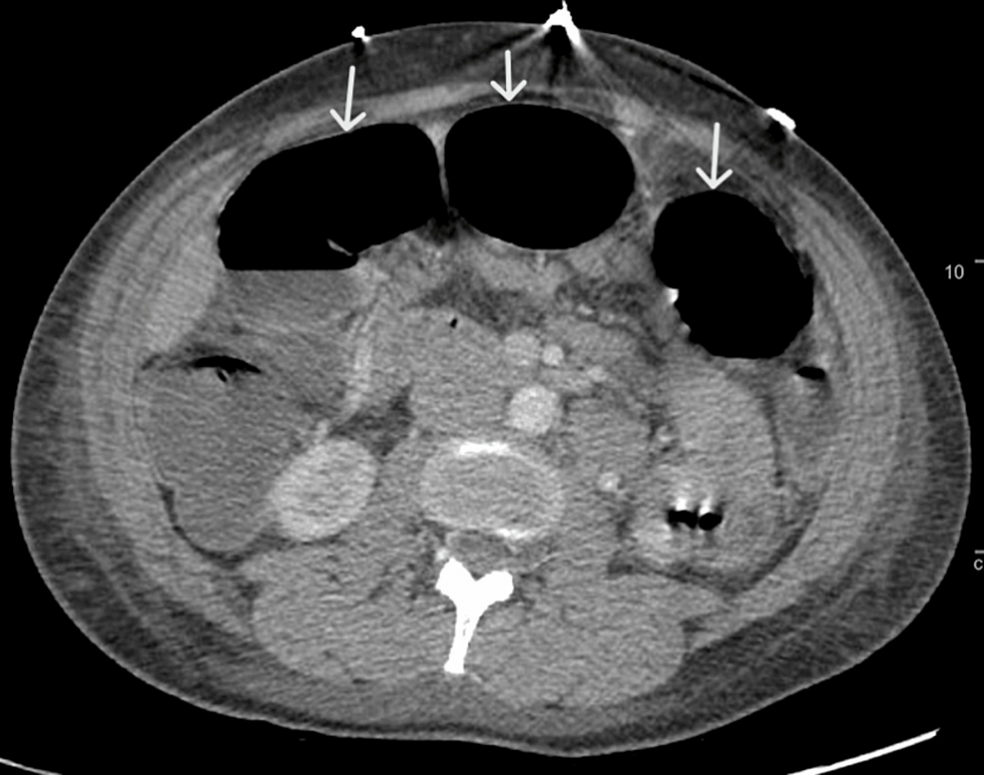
CT abdomen with contrast displayed dilatation of large bowel loops (white arrows) CT: computed tomography
